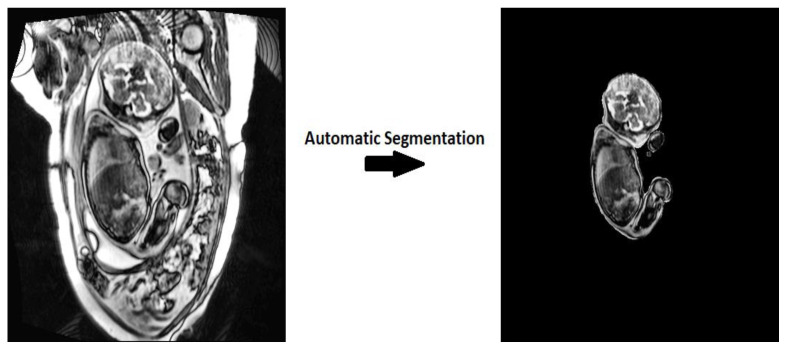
The given MRI dataset is applied through an automatic segmentation algorithm for clinical diagnosis.
